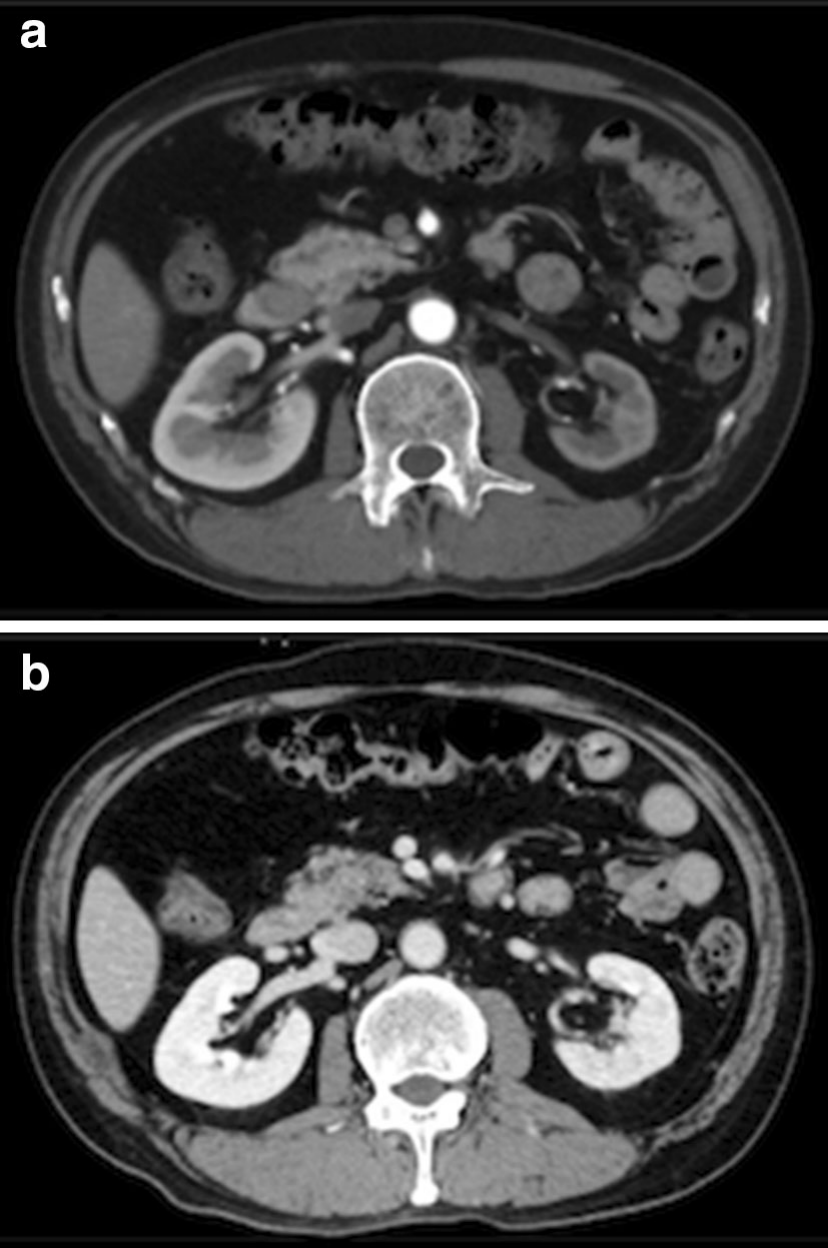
Computed tomography images of the kidneys demonstrating improvement in atrophy of the left kidney. a Before PTRA. b One year after PTRA
